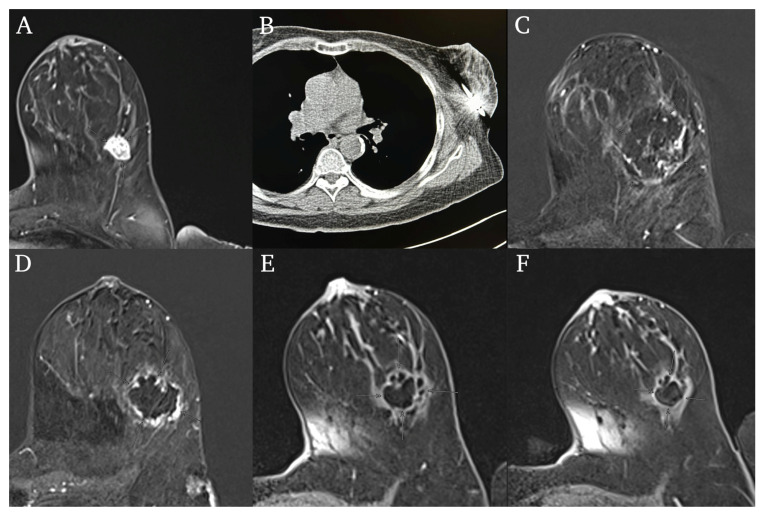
59-year-old female patient with cancer in the left breast. The Cryoablation was performed with two 9 min freeze cycles with a 9-min passive thaw cycle in between. (A) Pre-Ablation contrast-enhanced T1-weighted MR images. Tumor localized in left lower lateral quadrant. (B) The cryoprobe is inserted in the lesion during the CT-guided ablation procedure. (C) First post-ablation images, 24 h after ablation. The ablation zone can be seen in the contrast-enhanced T1-weighted MR images. (D) Ablation zone in the 3-month post follow-up MR-images. (E) Ablation zone in the 6-month post follow-up MR-images. (F) Ablation zone in the 9-month post follow-up MR-images. The patient showed a complete remission and had a survival time of 16 months starting at the date of ablation until last contact.
